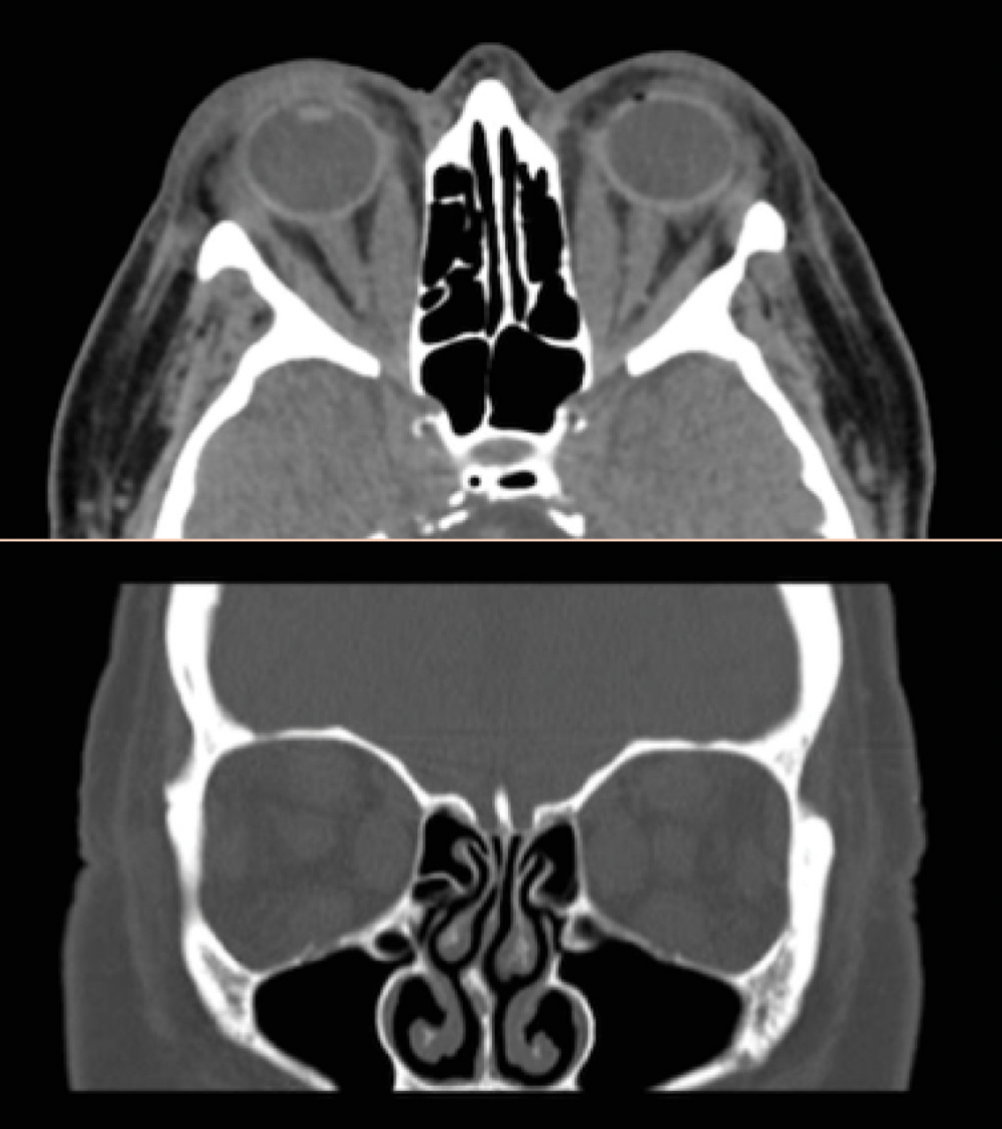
Transverse (upper) and coronal (lower) CT scan of the orbits with intravenous contrast showing expansion of the superior, medial and posterior recti in both orbits with significant bilateral proptosis.
